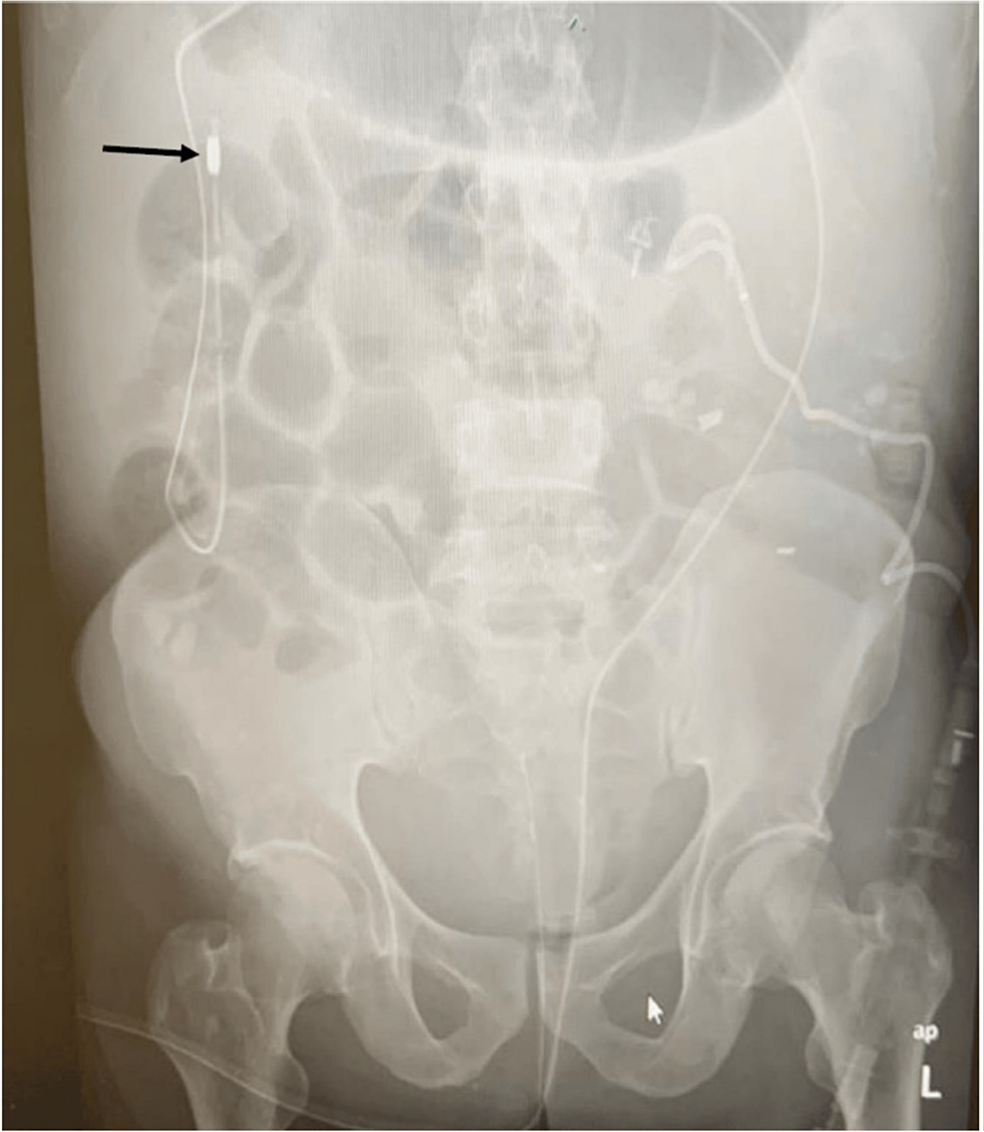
A post-procedure plain abdominal (AP supine view) X-ray. The image shows a significant interval improvement in the gaseous distention of the colon. The Ryle's tube is coiled up from the cecum, and the tip is in the hepatic flexure (line arrow).
